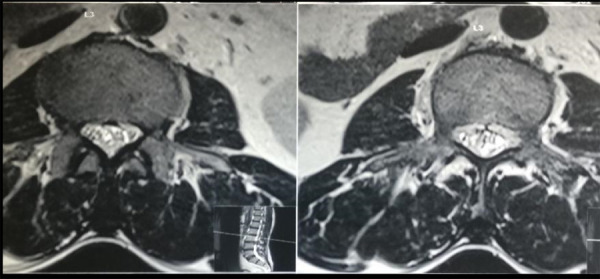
Preoperative 3 Tesla MRI LS spine T2 weighted axial section at the L_3_-L_4_ level.
